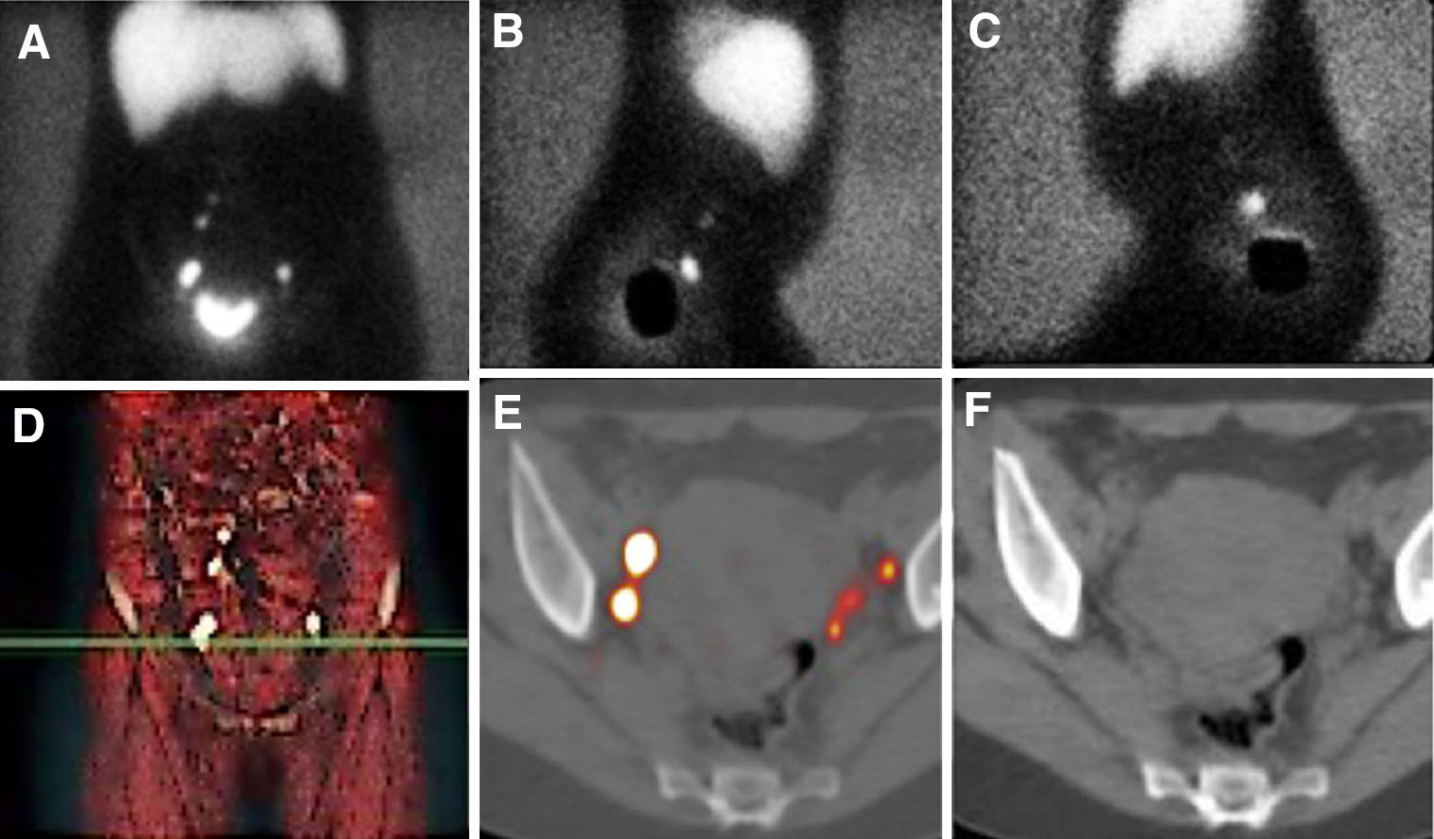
Cervical cancer. Planar images show a bilateral drainage in pelvic area (a–c). Volume-rendering image displays the level of sentinel nodes (d). SPECT/CT axial-fused images showing two separate nodes with high tracer uptake in right obturator fossa as well as three tiny nodes in left side (e). Corresponding axial CT slice (f)
